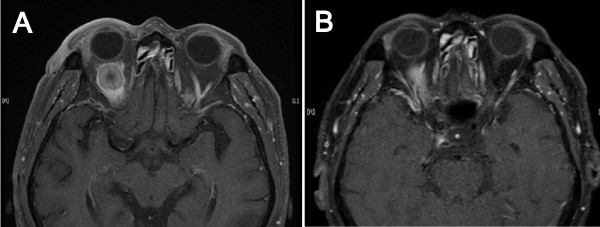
Brain magnetic resonance image.A: Showing an intra-orbital mass on the right side before gefitinib therapy. B: Revealing a reduction of the intra-orbital mass after two months of gefitinib therapy
